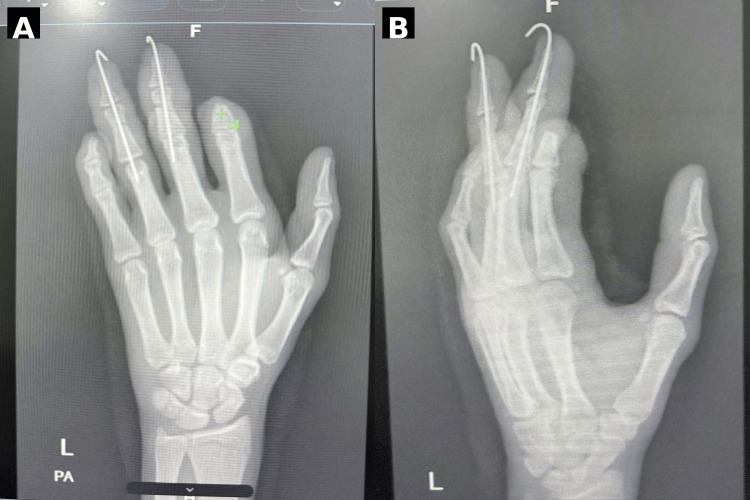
Postoperative radiographic evaluation (A) Anteroposterior (PA) view of the left hand demonstrating skeletal stabilization of the middle and ring fingers with Kirschner wires (K-wires). Length and alignment of the remaining phalanges are maintained. (B) Oblique view confirming satisfactory positioning of the K-wires and overall alignment of the digits after fixation.
